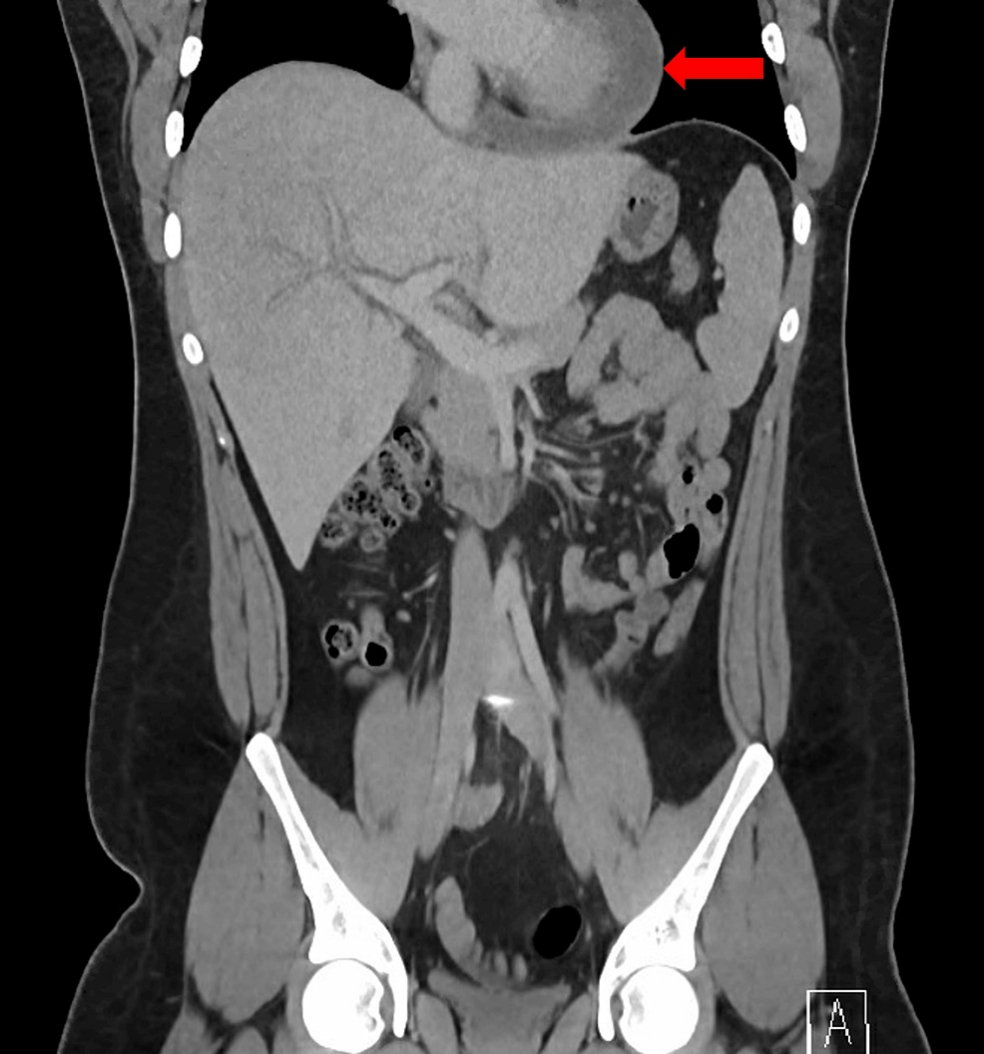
CT scan showing hepatomegaly, splenomegaly, and pericardial effusion (red arrow).
